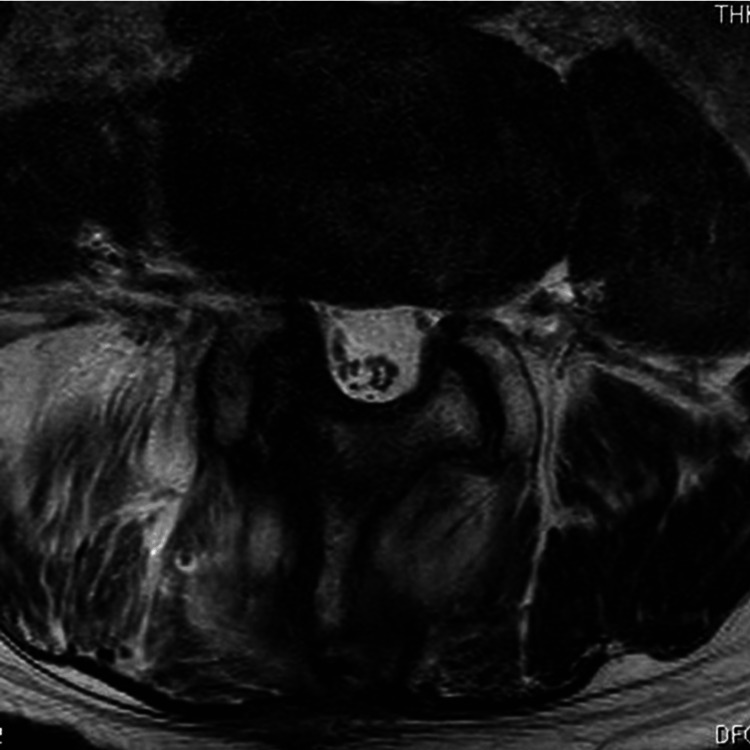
MRI at the L4–L5 facet level at three months after surgery. Axial T2-weighted MRI shows no hematoma recurrence
